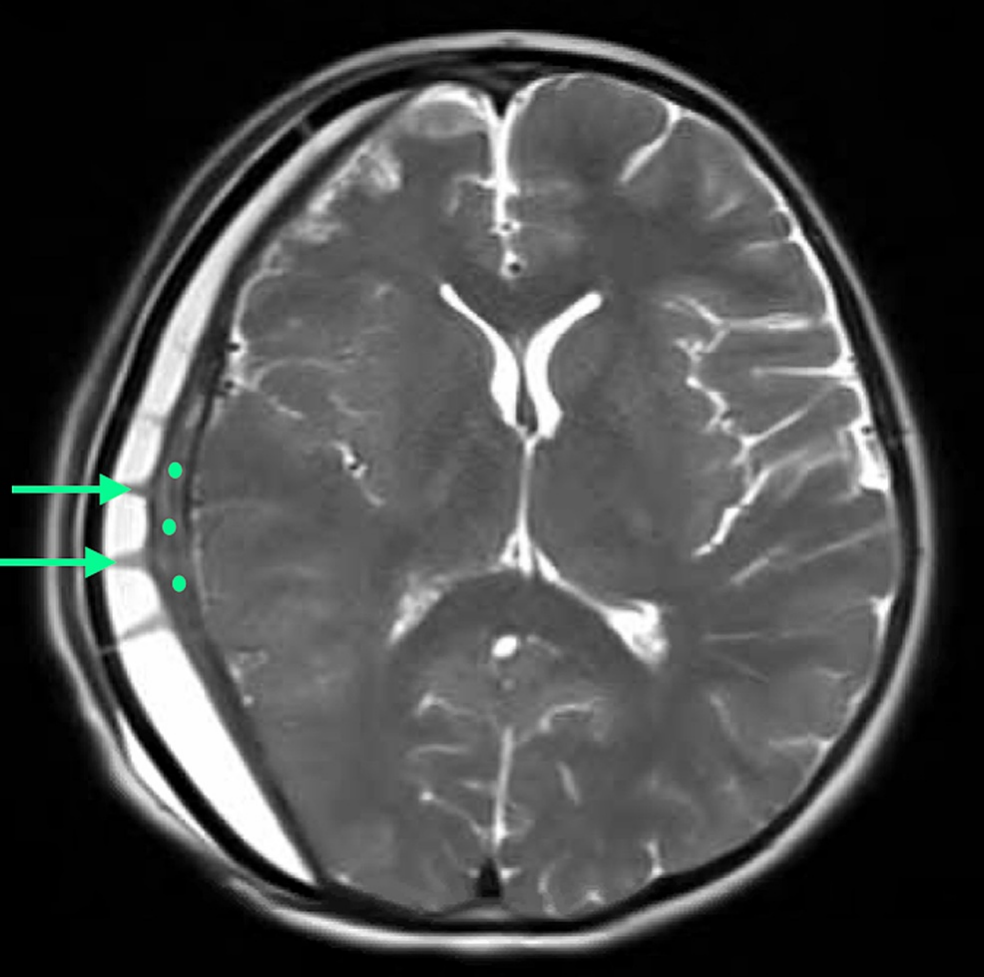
Brain MRI three months after the PEEK cranioplasty Limited brain MRI three months after the polyetheretherketone (PEEK) cranioplasty revealed a larger homogeneous epidural collection subjacent to the cranioplasty, with prominent columnar isointense structures (arrows) and a relatively thick and slightly fluctuant-appearing isointense layer on the epidural surface (dots).
